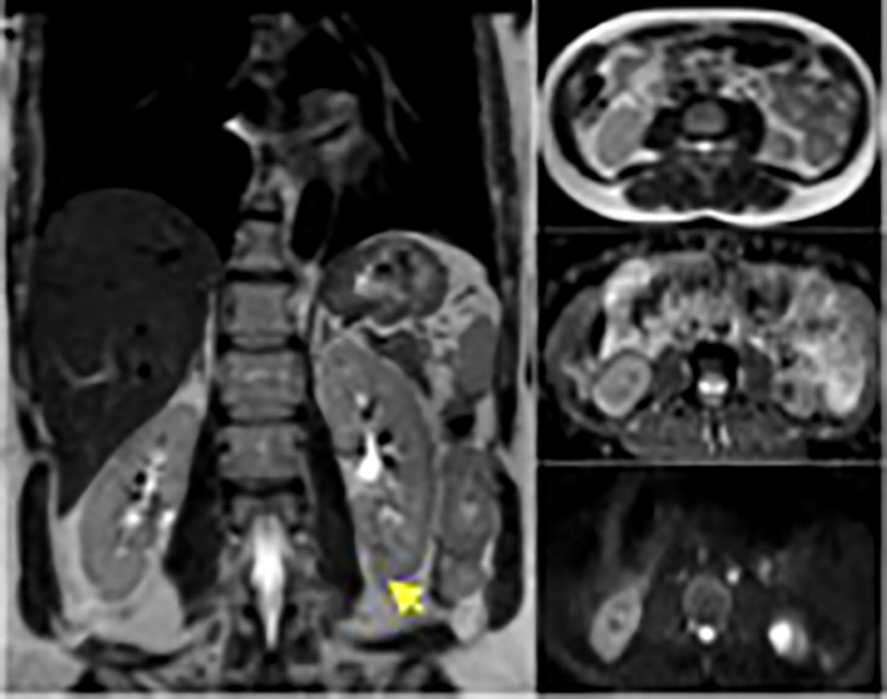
Enhanced MRI for the abdomen revealed solid enhancing left lower pole lesion with diffusion restriction.
